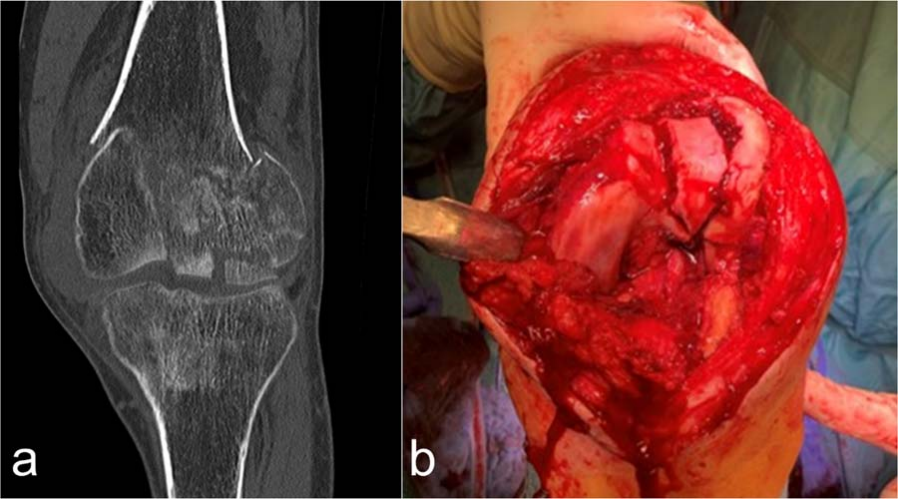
Case presentation of the knee region. (a) The coronal CT segment shows a transcondylar multifragment fracture of the femoral bone. (b) The intraoperative situs shows the comminuted zone of the condylar articular surface.
